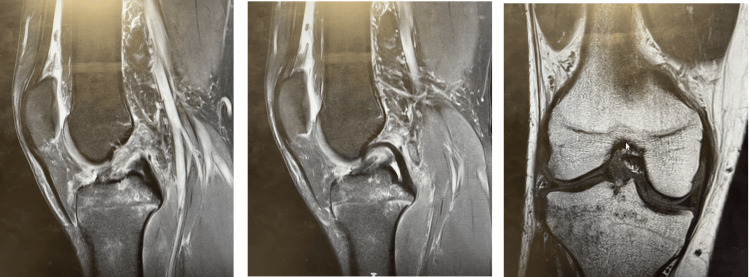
Sagittal and coronal magnetic resonance imaging (MRI) scans of a 34-year-old male patient showing torn anterior cruciate ligament (ACL).
